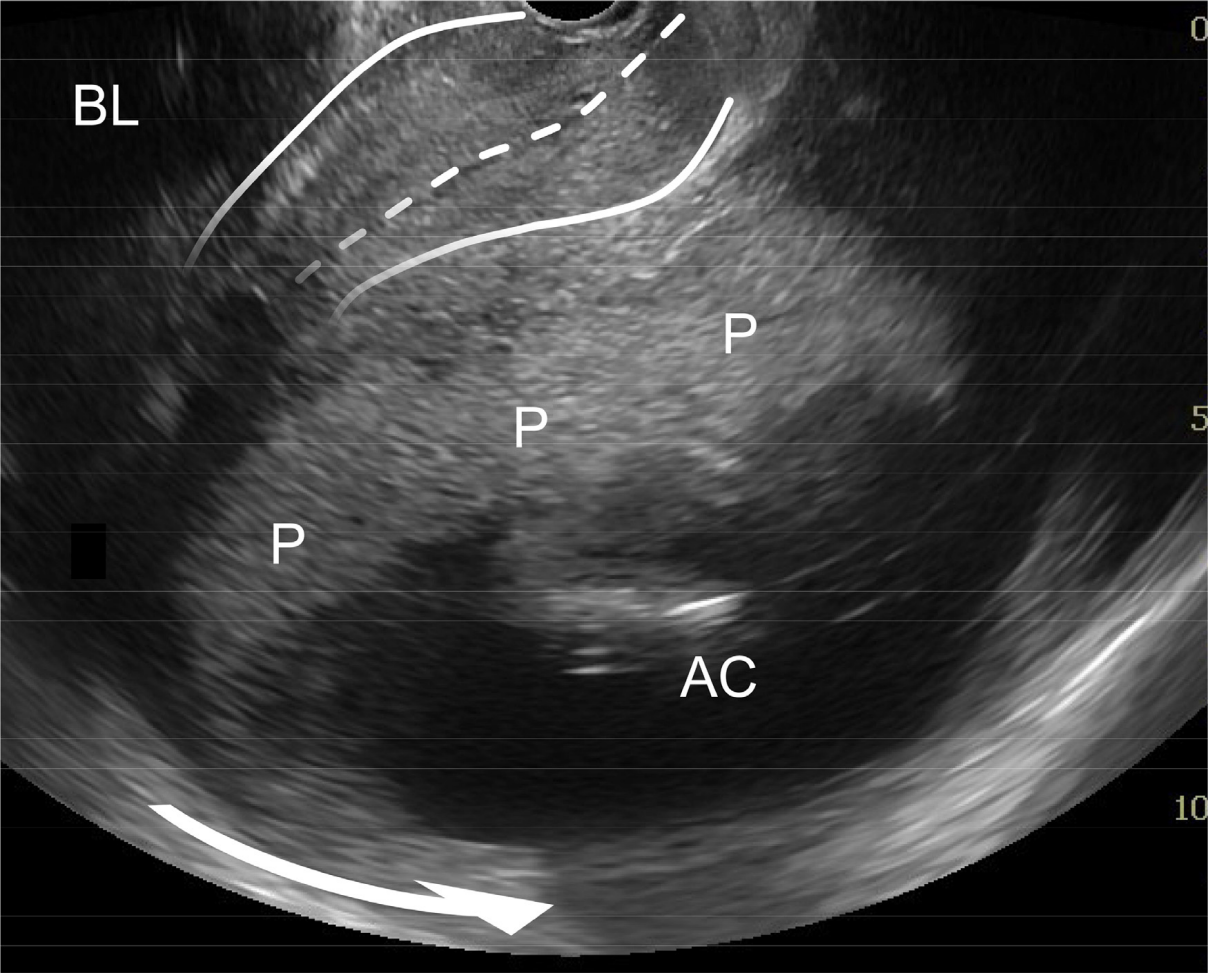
Transvaginal ultrasound at 16 weeks of gestation Transvaginal ultrasonography showing an elongated cervix (*solid* and *dotted lines*) and significant cranial displacement of the internal cervical os above the symphysis pubis caused by a retroflexed incarcerated gravid uterus (*arrow*). The placenta is attached to the anterior wall. However, determining the positional relationship between the internal cervical os and the placenta is difficult via transvaginal ultrasonography because of the elongated cervix, which complicates the diagnosis of placenta previa. *AC*, amniotic cavity; *BL*, bladder; *P*, placenta.
